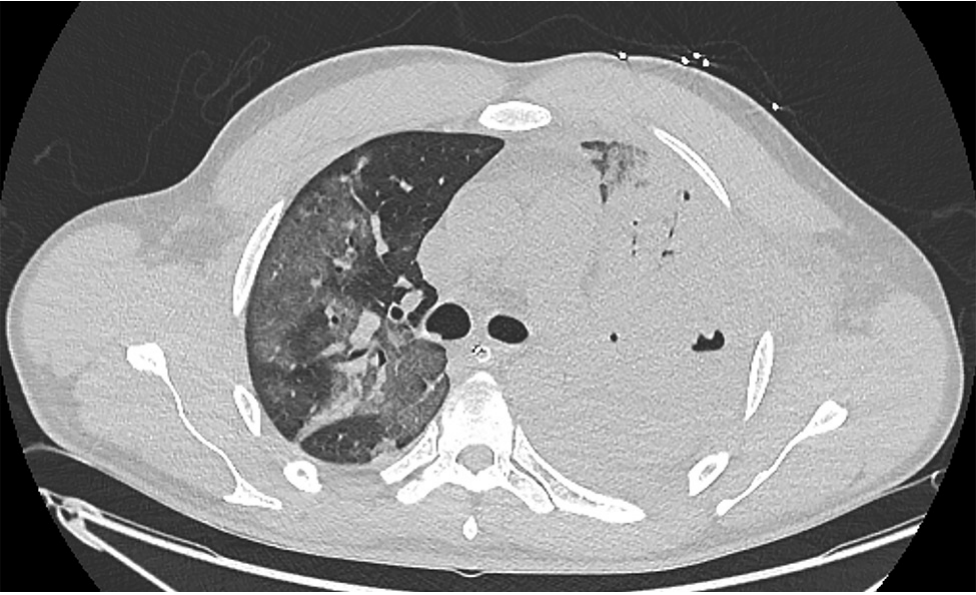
Chest computed tomography of a patient in France with Panton-Valentine leukocidin–secreting *Staphylococcus aureus* pneumonia complicating coronavirus disease, showing worsening of bilateral parenchymal damage with complete consolidation of the left lung, cavitary lesions suggestive of multiple abscesses, and appearance of areas of ground-glass opacities in the right lung
